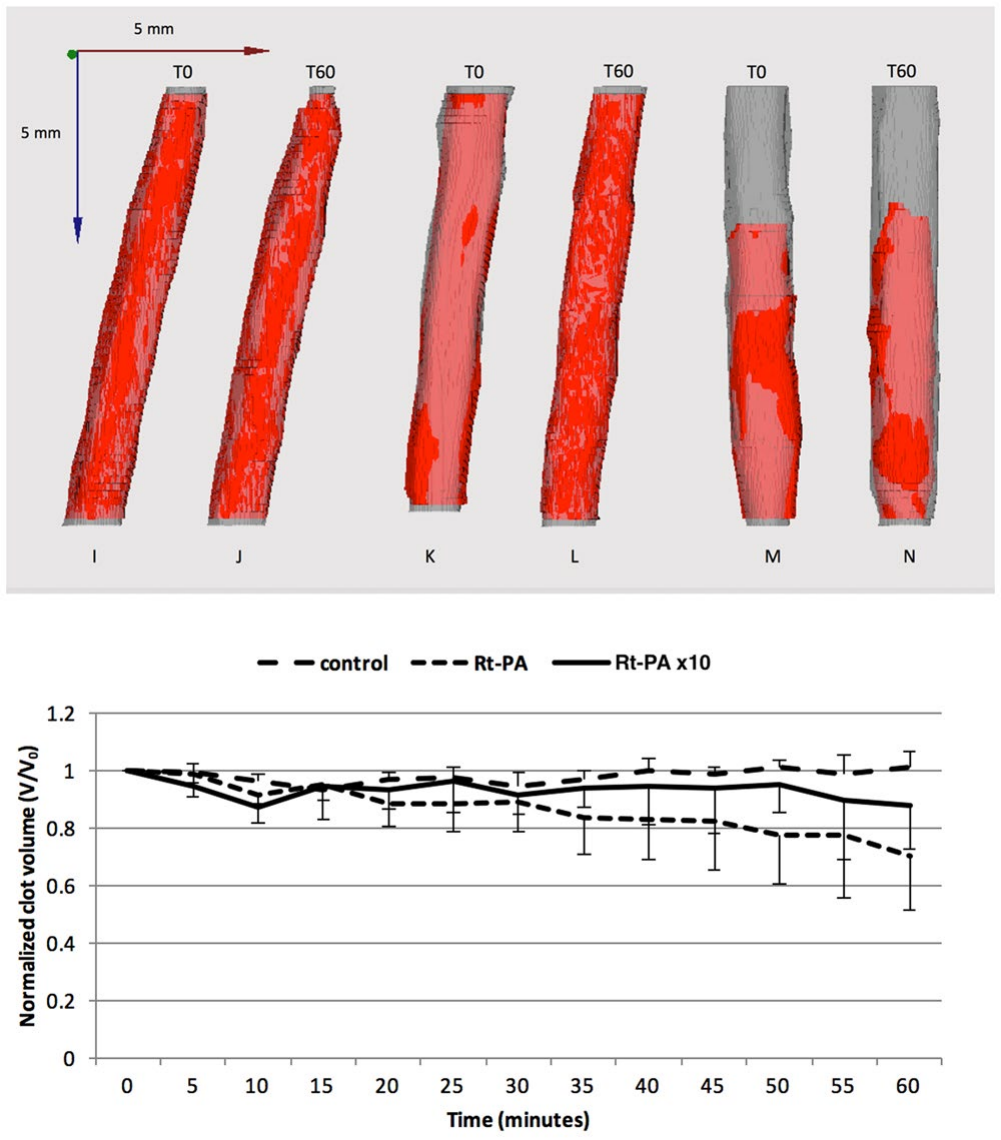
In vitro* Rt-PA delivery in the full occlusion model. Blood clots were treated with a range of Rt-PA alone in a range of concentrations (0, 3, 30 µg/ml). The blood clot volume was assessed every 5 min for 60 min using high frequency ultrasound imaging. 3D reconstructions of clots were made at T0 and T60 (top). I-J: Control; K-L: Rt-PA; M-N: Rt-PAx10. Data expressed as mean ± SEM were calculated from four independent experiments (bottom).
